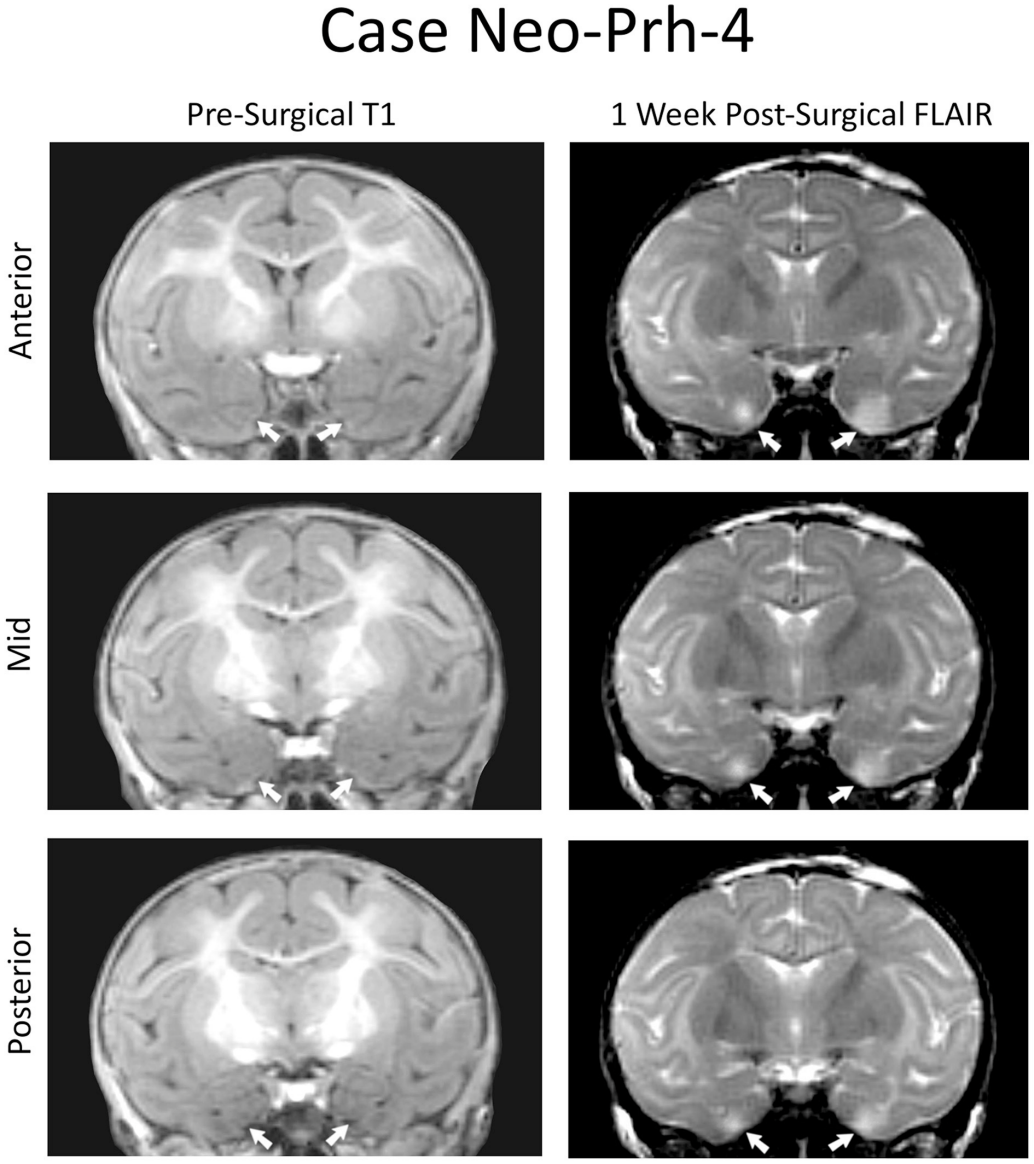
Coronal MRI from a representative case (Neo-PRh-4). Pre-surgical structural T1-weighted images at three rostro-caudal levels through the perirhinal cortex (left column). Post-surgical FLAIR images (right column) at the same rostro-caudal levels show hypersignals (whiter areas) that are indicative of edema and cell damage. Arrows point to the rhinal sulcus on the left and to hypersignals on the right.
